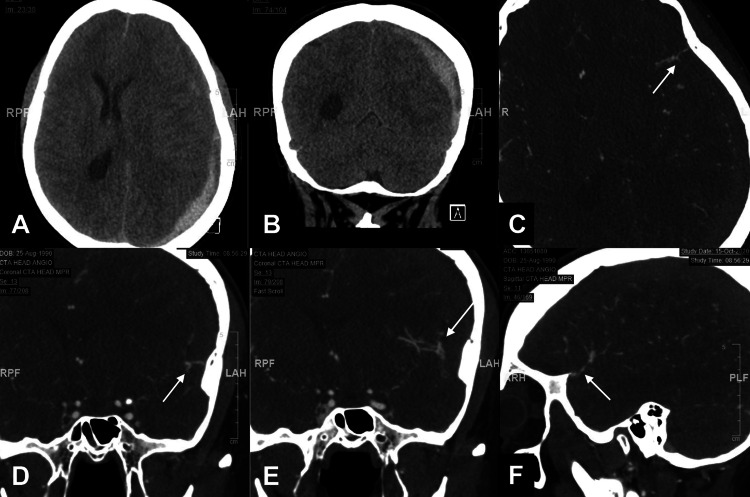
Non-contrast CT and CT angiogram images. A: Non-contrast axial and B: Coronal head CT, approximately seven hours after initial imaging, demonstrating a left holohemispheric, mixed density subdural hematoma with layering hyperdense blood products and approximately 1.1 cm of midline shift. CTA showing C: Axial, D: Coronal, E: Coronal, slightly more posterior compared with D; and F: Sagittal, capturing a prominent superficial cortical vasculature crossing over the hematoma as a potential etiology for the spontaneous subdural hemorrhage. White arrows highlighting location of presumed vascular abnormality.
